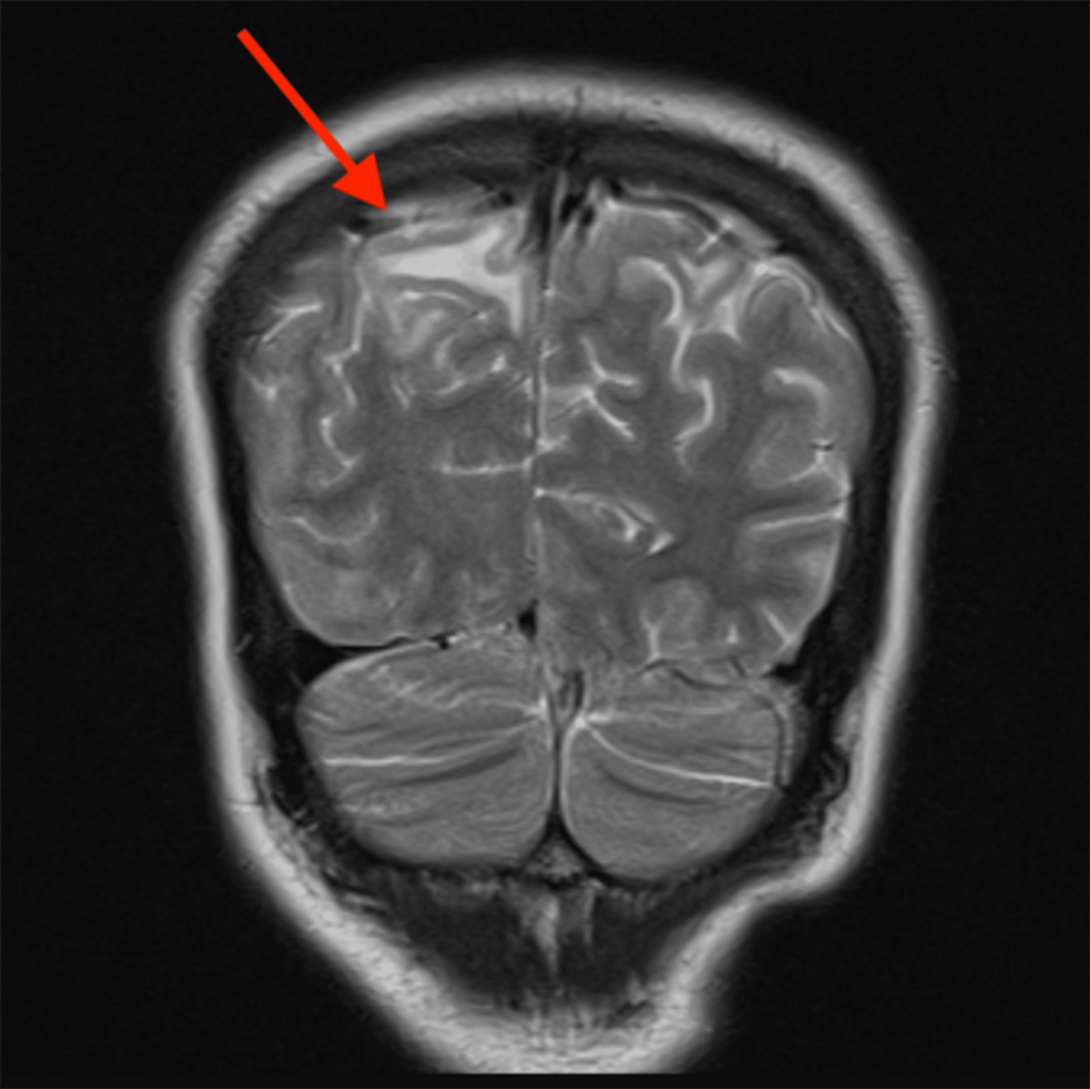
Coronal T2 MRI of Patient 2 (MN) showing hyperintensity (arrow) in the parietal region The red arrow points to the parietal region with patchy, symmetrical cortical and subcortical T2 hyperintensities, consistent with PRES MRI: magnetic resonance imaging; PRES: posterior reversible encephalopathy syndrome
